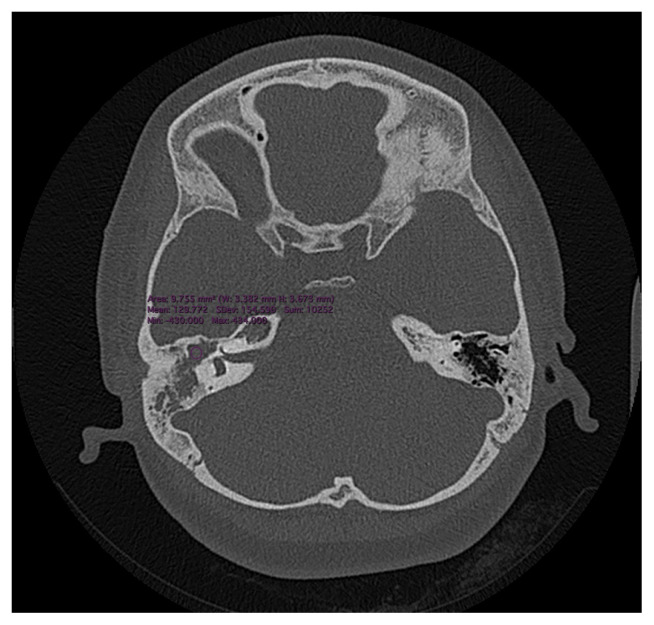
In the axial plane section of the 34 years old male cholesteatoma (CHS) patient’s non-contrast temporal bone high resolution computed tomography (HRCT), the region of interest (ROI) with pathological soft tissue density was hand-marked at the epitympanum level, and its transfer to an XML file is displayed.
